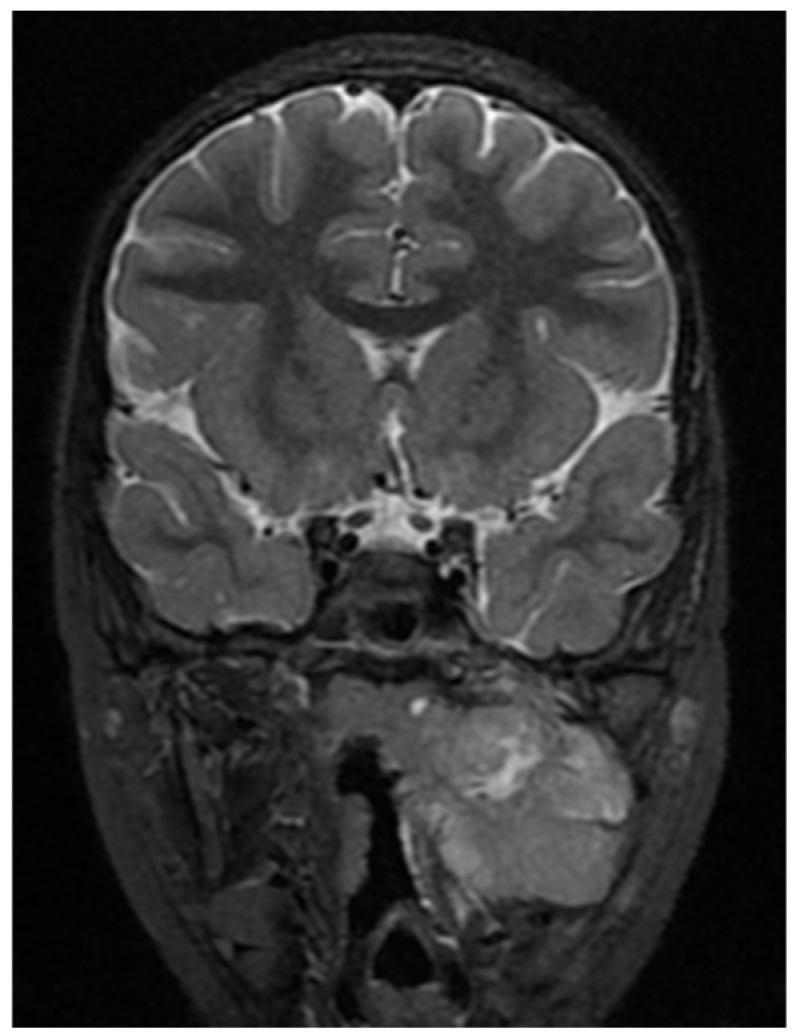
MRI of the patient on postoperative day 17.
